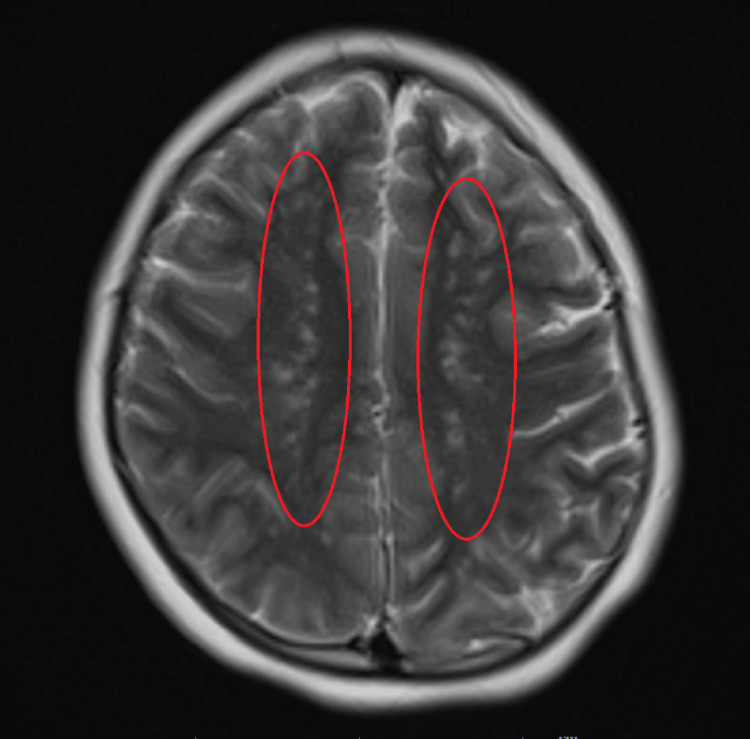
Axial diffusion-weighted MRI showing innumerable foci of bright diffusion (red circles), demonstrating bilateral supratentorial watershed infarcts in the ACA-MCA border zones consistent with hypoperfusion injury MRI: magnetic resonance imaging, ACA-MCA: anterior cerebral artery-middle cerebral artery
